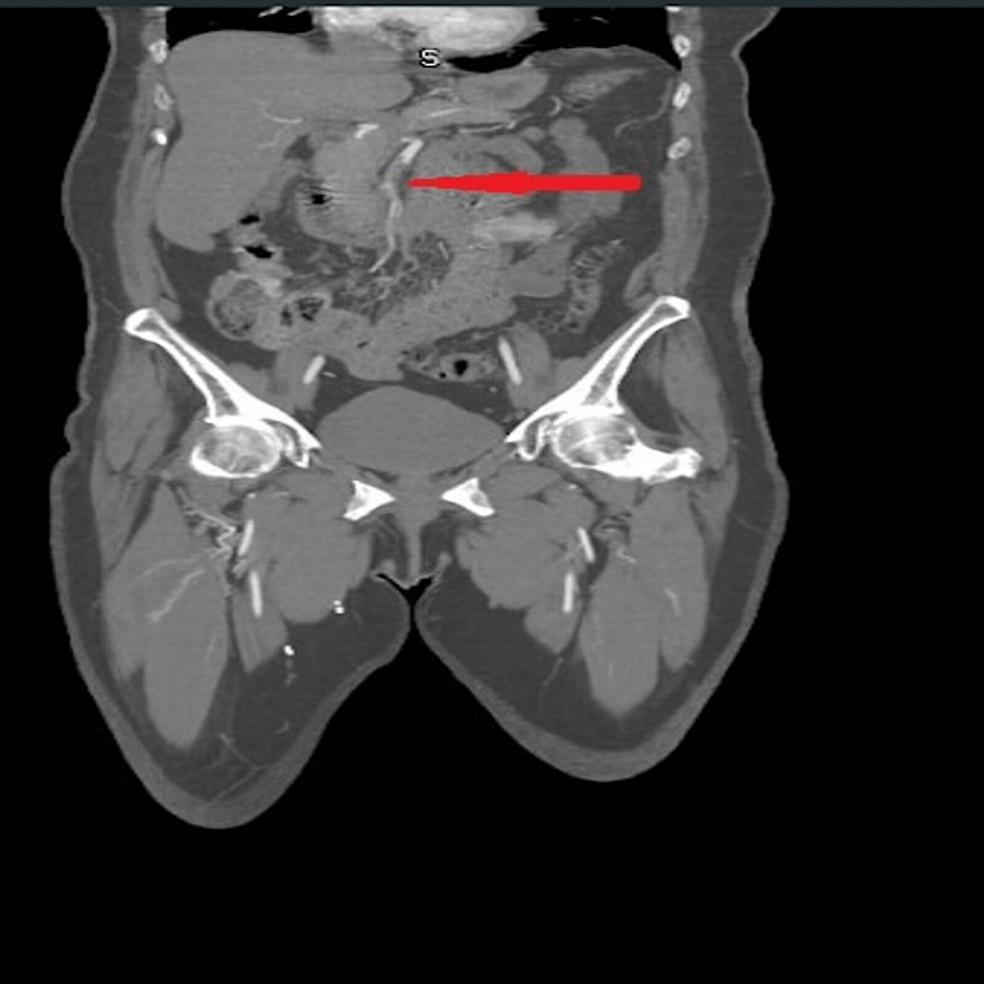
CT of abdomen and pelvis showing thrombosis of superior mesenteric artery
